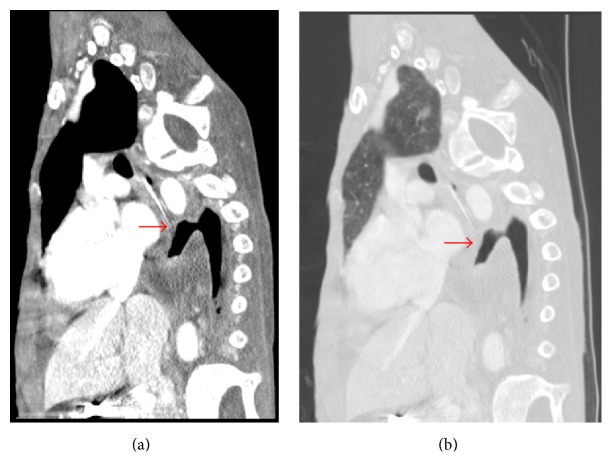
Chest CT: (a) mediastinal window, (b) lung window. Sagittal view showing esophageal tear and communication with pleura (red arrow).
